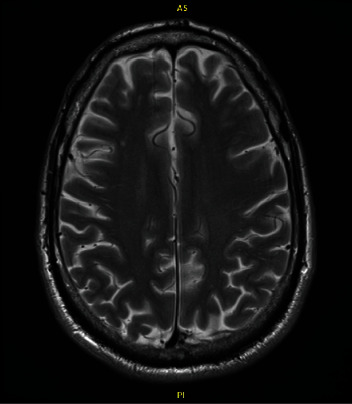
Follow-up MRI showing resolution of SDH.
